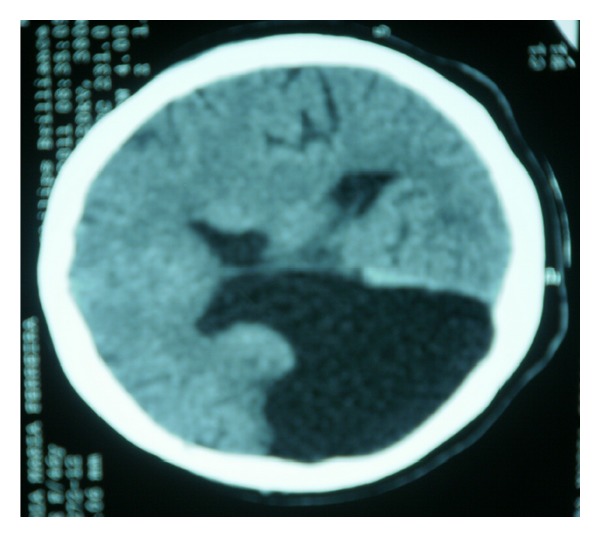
Head CT scan (October 4, 2011).
